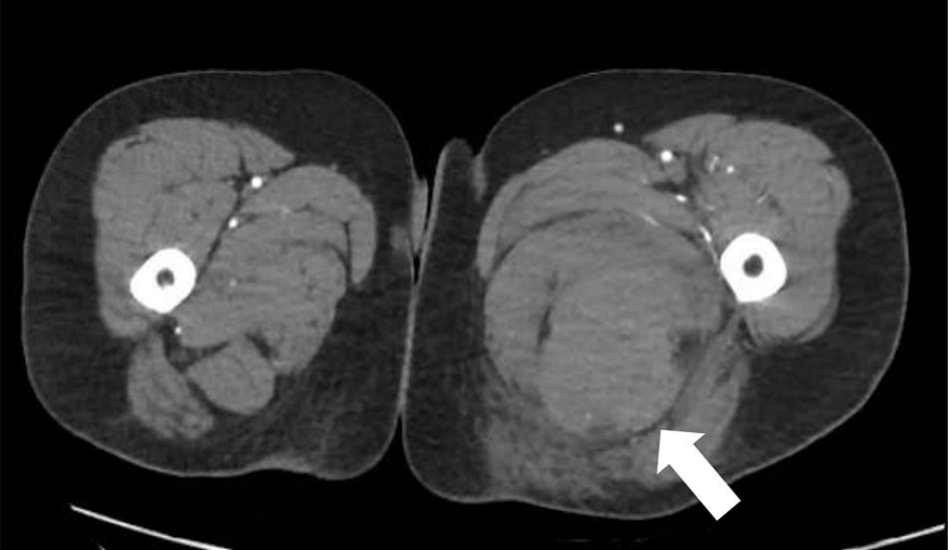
CT angiography axial slice demonstrating haematoma in posterior compartment of left thigh (white arrow).
